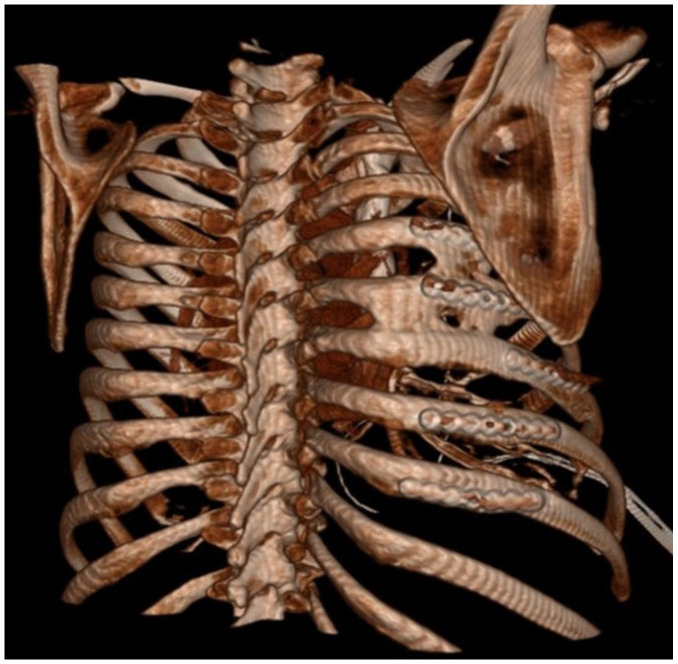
Three-dimentional reconstruction of CT scan with HO between ribs.
